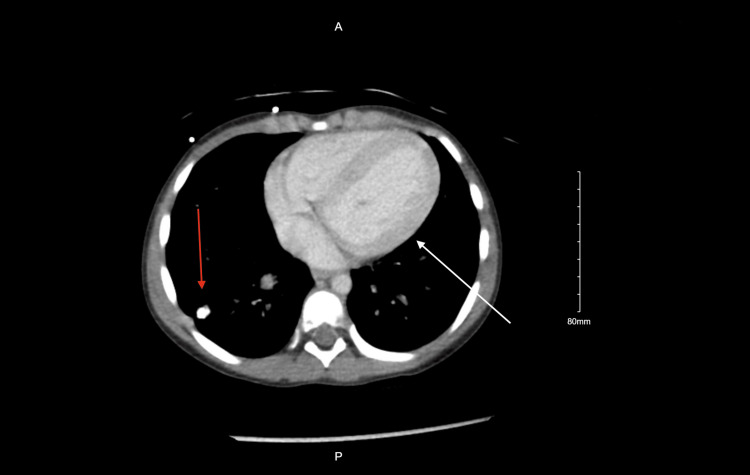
Contrast enhanced CT of the chest showing mild cardiomegaly and left ventricular dilation (white arrow) as well as a right lung base granuloma (red arrow)
